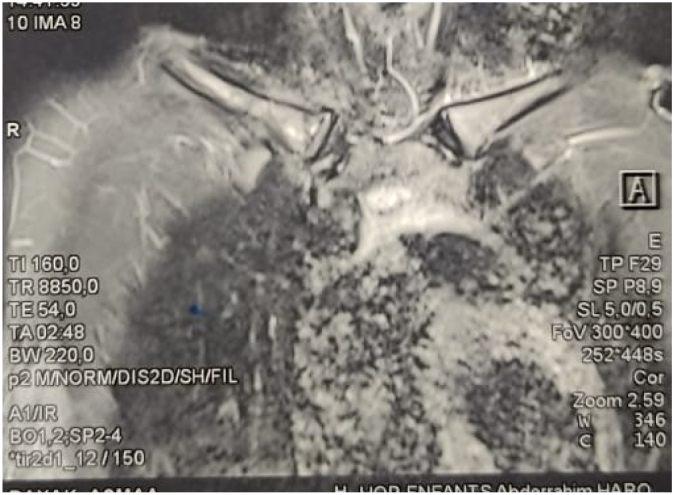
MRI image of the sternal end of the clavicle showing an abnormal signal from the sternal manubrium.
